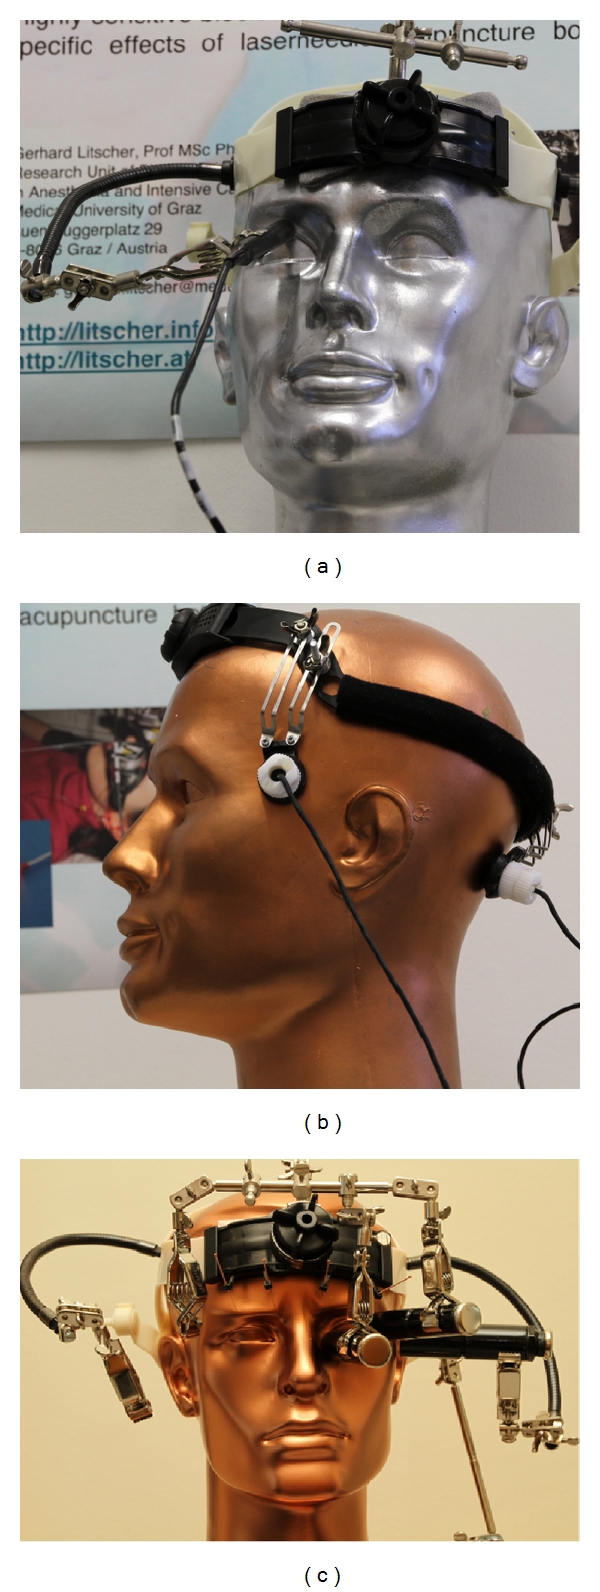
“Windows to the brain” for transcranial ultrasound investigations: transorbital (a) and (c), transtemporal ((b) left, (c) right) and transnuchal ((b) right). The probe holder constructions were developed at the TCM Research Center in Graz, Austria.
